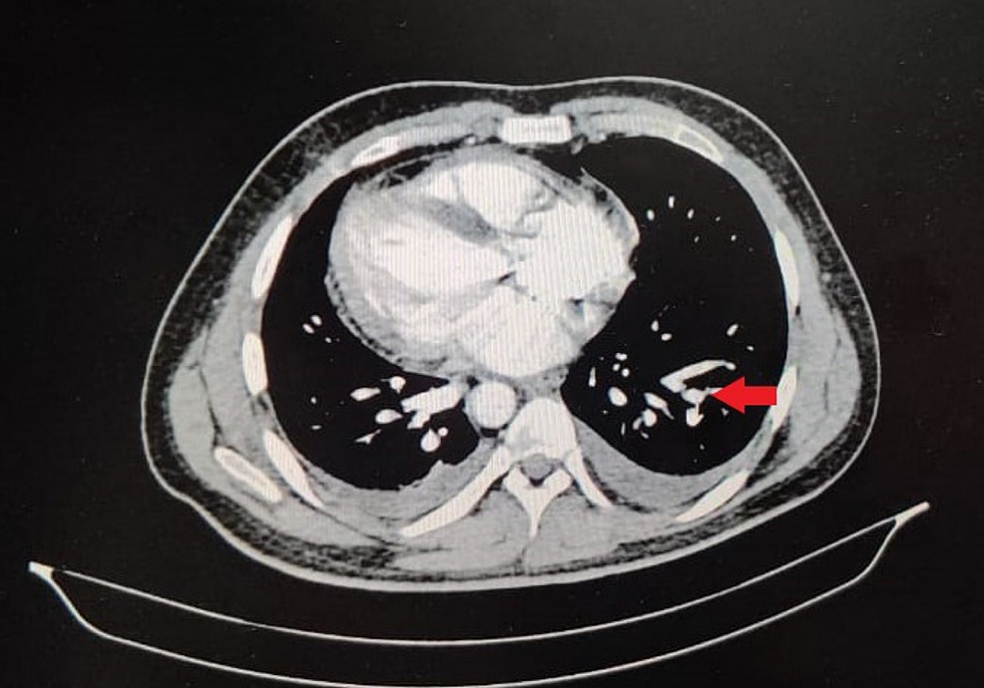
CT pulmonary angiography showing the presence of the sub-segmental embolus
